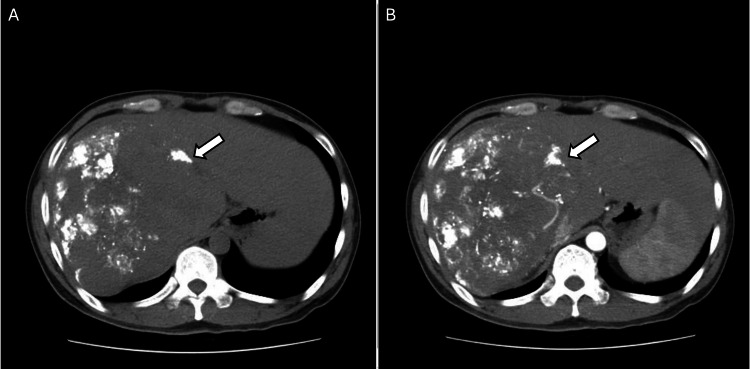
Contrast-enhanced computed tomography (CT) images obtained before embolization. A) Pre-contrast axial CT image showing the residual tumor (arrowhead) in the right hepatic lobe. (B) Post-contrast axial CT image demonstrating enhancement of the residual tumor (arrowhead), which is supplied by the right hepatic artery and the right inferior phrenic artery.
